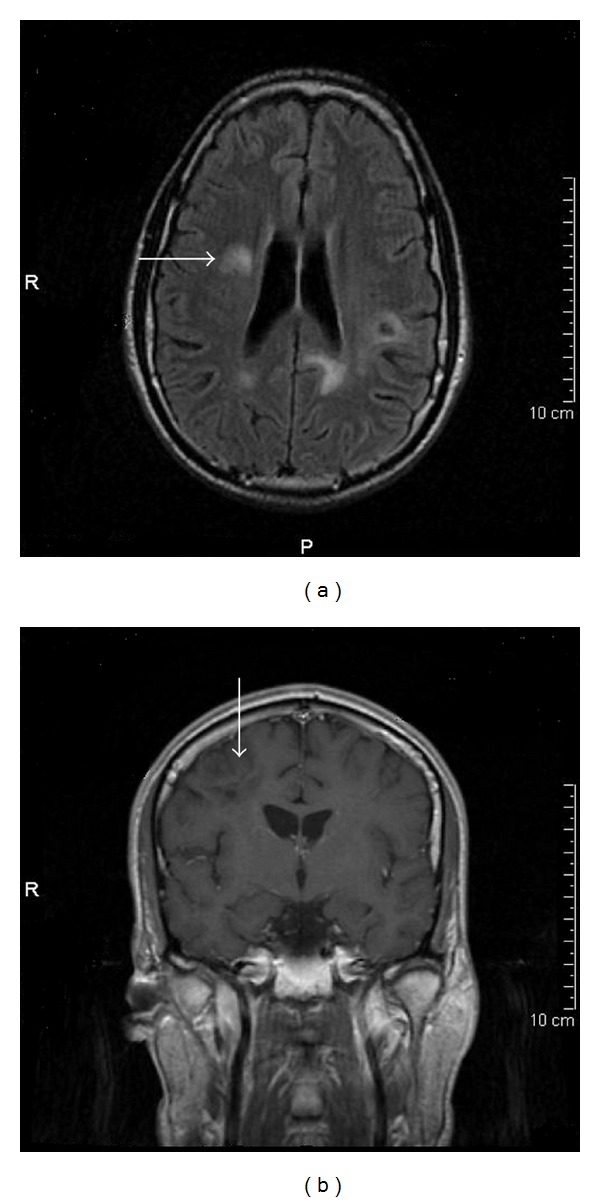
MRI scan after WBRT. After radiation therapy axial FLAIR image shows marked-size reduction of right-frontal mass (a) with no contrast enhancement in T1-weighted image after administration of gadolinium (b). According to International Revised Criteria for PCNSL Response Assessment, it was considered a complete response. Subsequent follow-up scans did not display any changes on remaining lesions and a FDG-PET scan undergone after completion of immunochemotherapy confirmed no residual tumoral activity.
